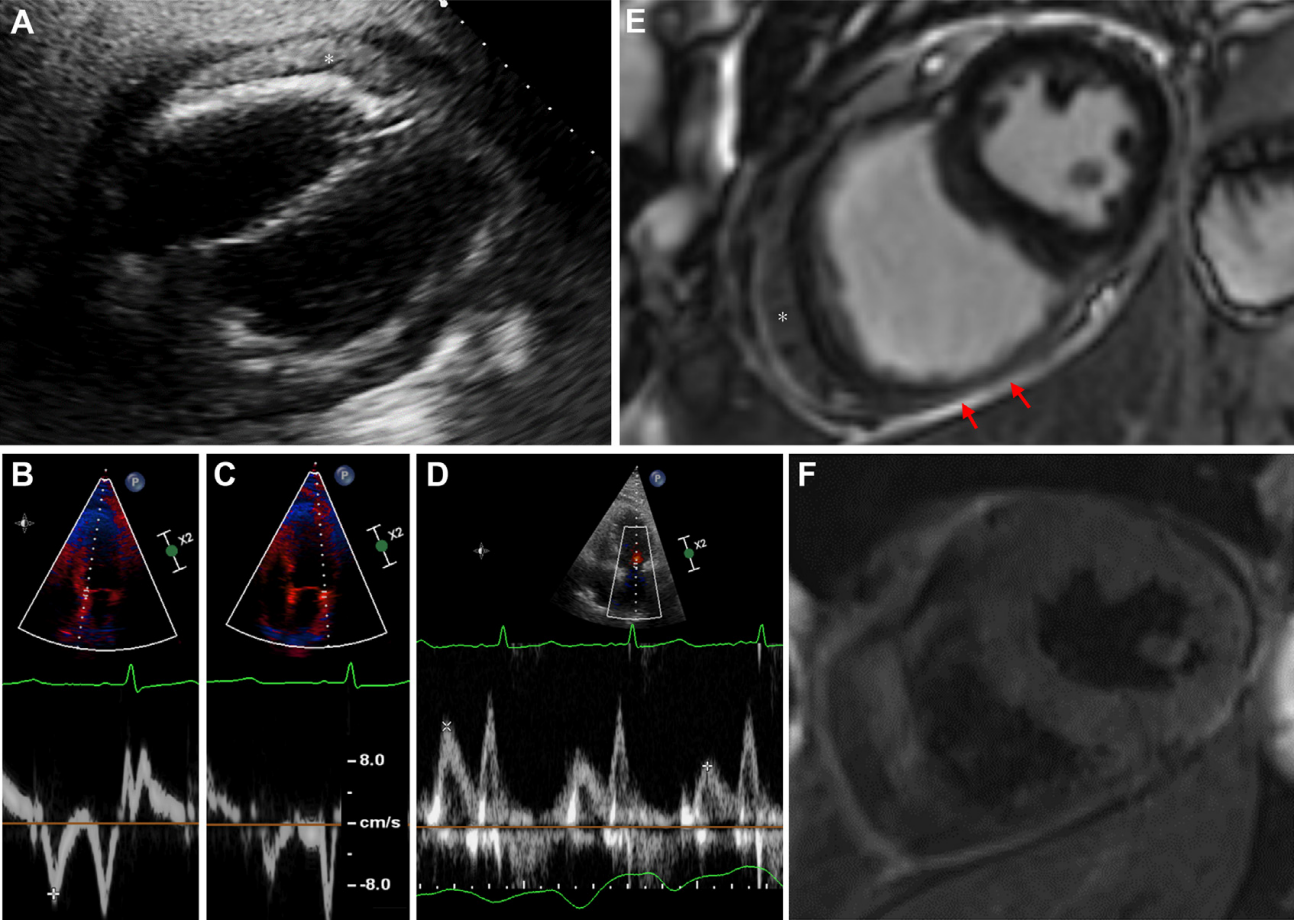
Imaging Findings of Patient 1 (A) Transthoracic echocardiography subcostal view displays moderate circumferential pericardial effusion without diastolic compression of right heart cardiac chambers. A prominent epicardial fat pad (∗) is noted adjacent to right ventricular free wall. (B) Septal mitral annular tissue Doppler velocity (e'_med_ = 9 cm/s) exceeding the (C) lateral mitral annular tissue Doppler velocity (e'_lat_ = 7 cm/s), demonstrating annulus reversus. (D) Pulsed wave Doppler shows significant respirophasic variation in mitral inflow velocity (E_exp_ = 69 cm/s, E_insp_ = 38 cm/s, percentage velocity change = 45%). (E) Cardiac magnetic resonance images 15 days after admission display pericardial thickening and delayed gadolinium enhancement on phase sensitive inversion recovery sequences (red arrows). (F) T2 black blood sequences demonstrate bright signal of the pericardial layers consistent with pericardial edema.
